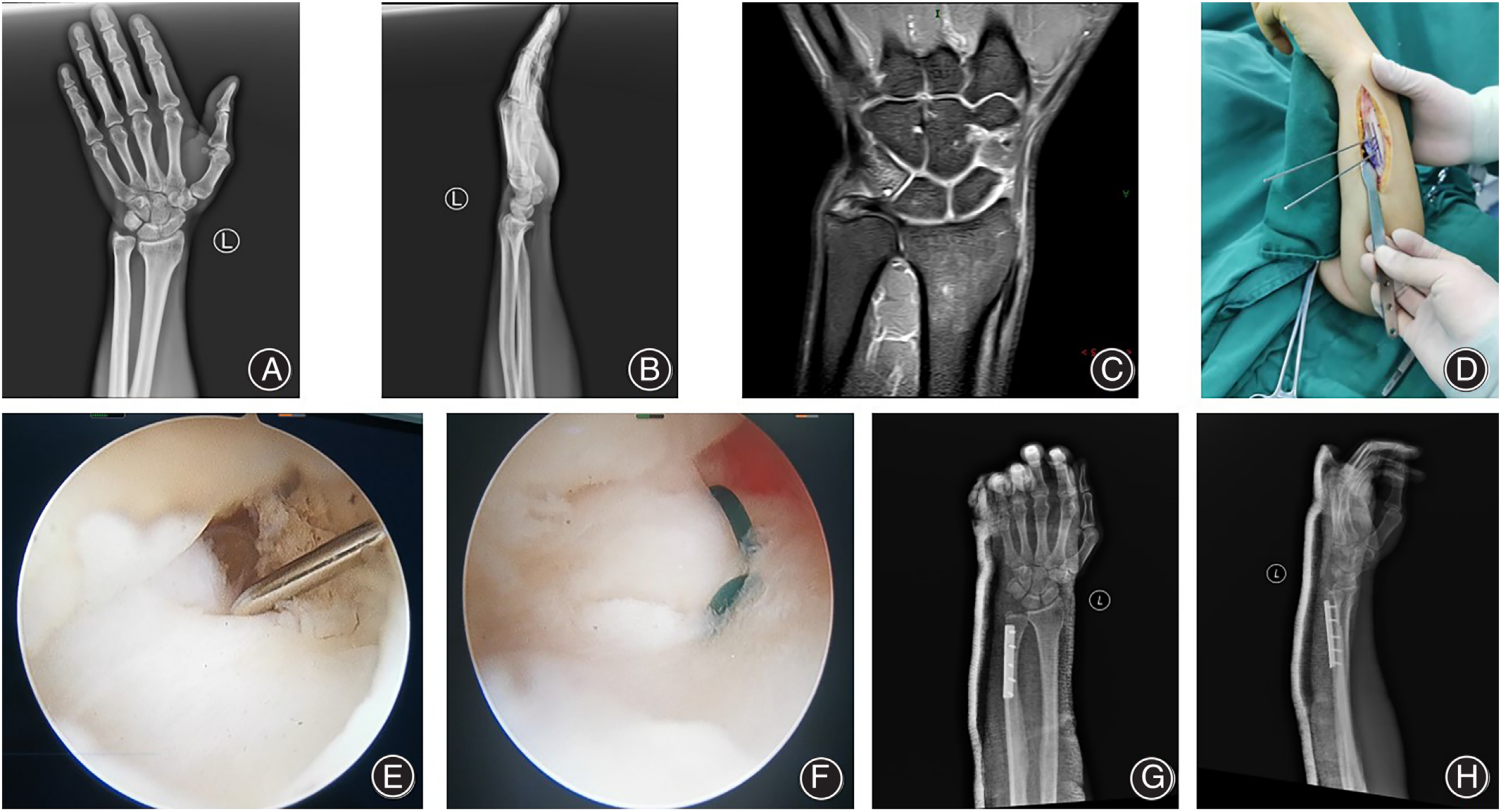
Typical Case 2. Male, 39 years old, with no obvious cause of left wrist pain and limited movement for 2 years, aggravated for 4 months, diagnosis of left wrist UIS, TFCC injury. Treated by wrist arthroscopy combined with oblique USO. (A, B) Preoperative anterolateral X‐ray films showed positive ulna variation; (C) Preoperative MRI showed positive ulnar variation combined with TFCC injury; (D) Intraoperative oblique USO. (E, F) Intraoperative suture of TFCC; (G, H) Postoperative X‐rays showed that the ulnar osteotomy was well‐aligned, and the ulnar positive variation had recovered
